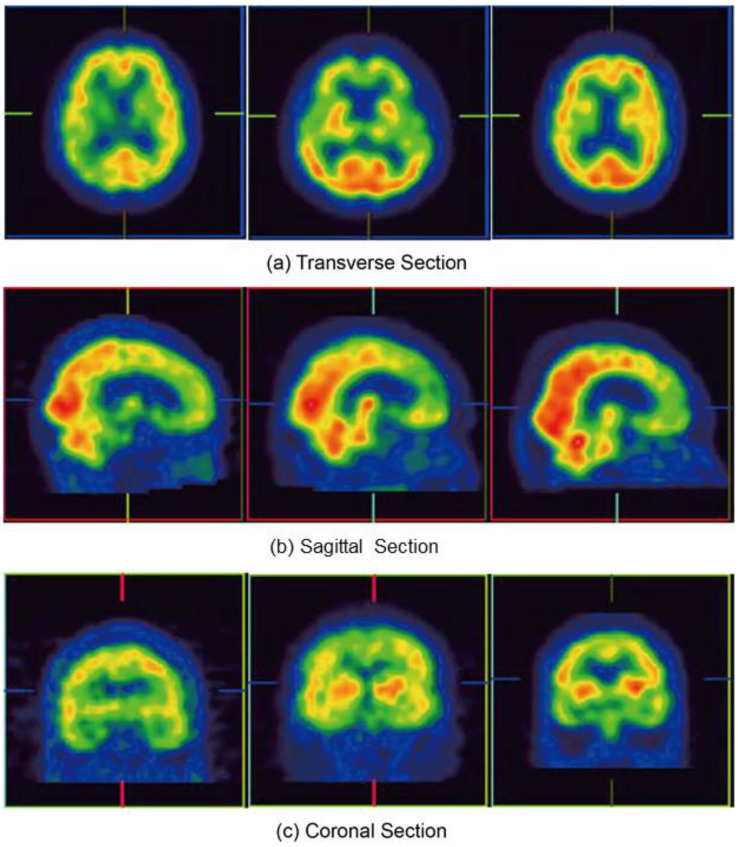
Three sections of SPECT images. (a) Transverse section; (b) Sagittal Section; (c) Coronal section. The colors in the figure represent the ranges of blood flow with red being the most blood flow and violet the least.
